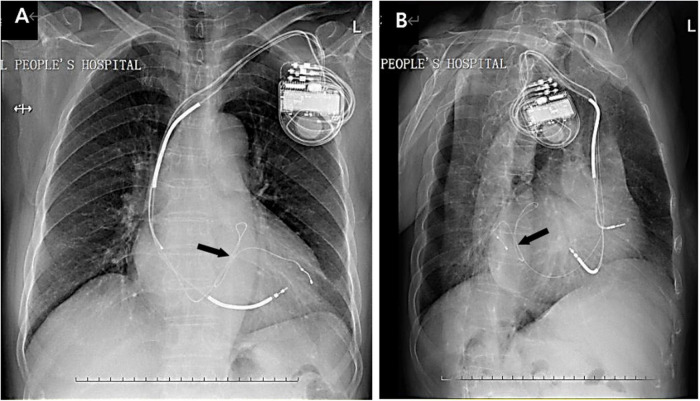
Chest X-ray images from anteroposterior projection (A) and lateral projection (B) show the left ventricular lead implanted through the coronary sinus by the loop technique at the 12-month follow-up. The arrows show the loop in the location of the coronary vessel.
